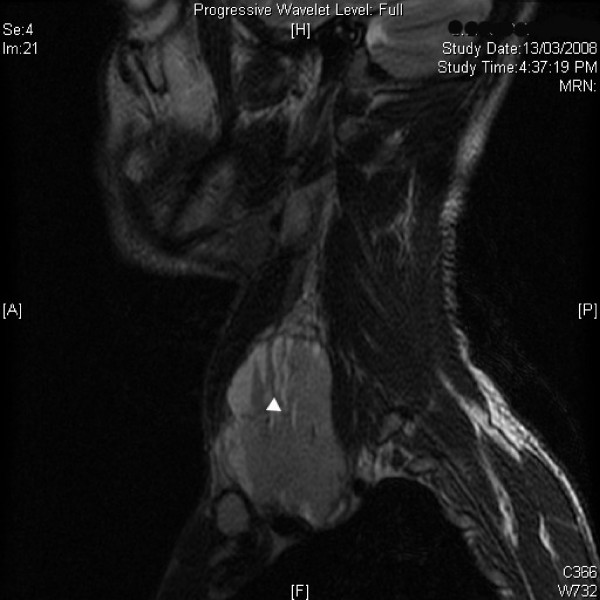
T2 W magnetic resonance image of the neck (sagital section) showing hyperintense mass with fluid-fluid levels (arrow head, pointing upwards).
